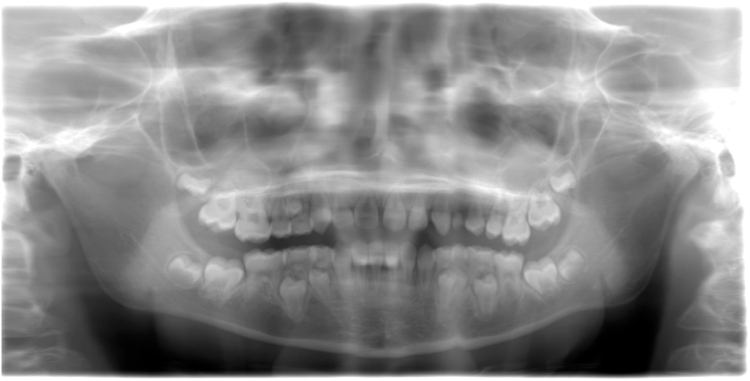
POIKTMP is associated with dental anomalies. Orthopantomogram of F5 at age 11 years and 11 months, showing short roots on all teeth, microdontia on 14/24, severe molar incisor hypomineralisation (MIH) on 16, mild MIH on 26/36/46/31/32/21, reduced overlap and overhang, inverted dental articulation on 32/22 and diastema on 11/21.
